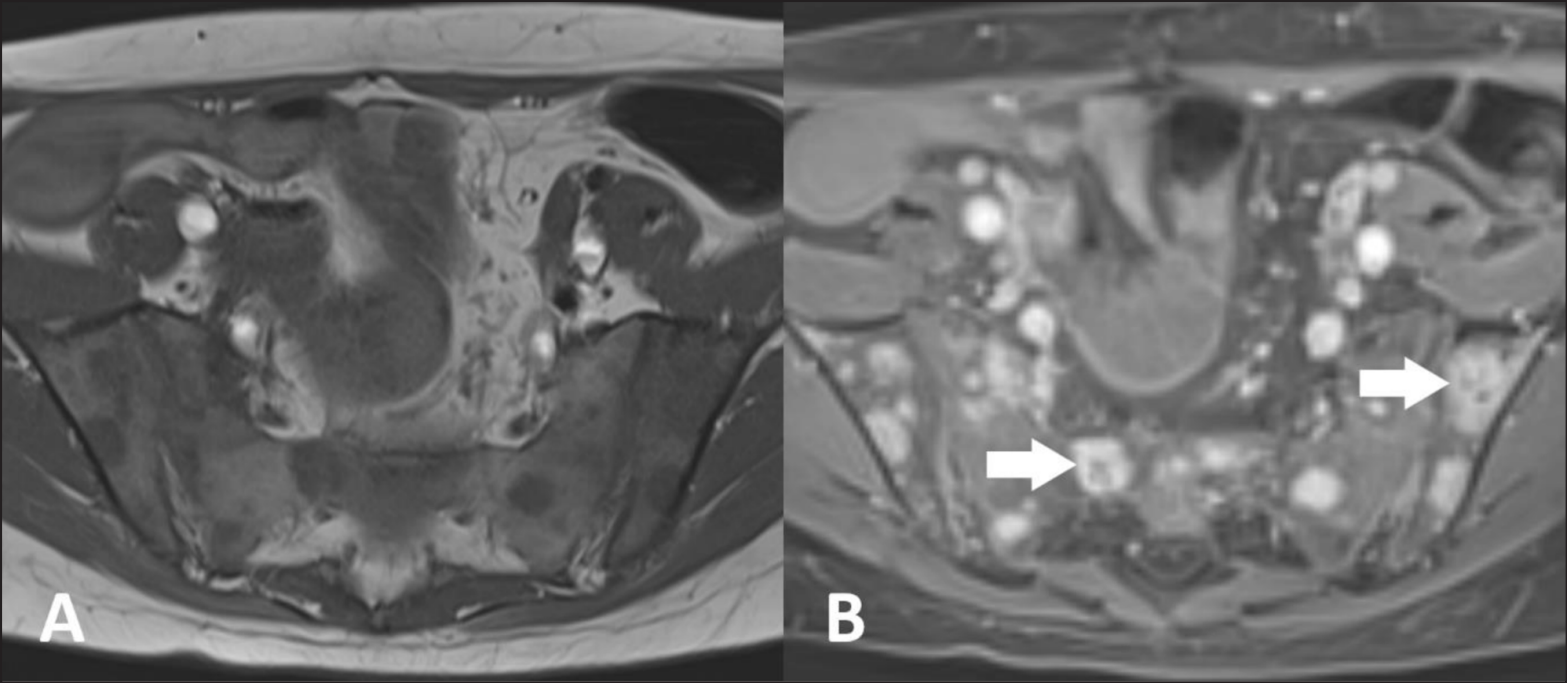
A 13-year-old female with hemangiosarcoma and sacral metastasis. (a) Axial T1-weighted MR-image shows multiple hypointense lesions in sacral and iliac bone. (b) Axial fat-saturated post-contrast T1-weighted MR image shows strong enhancement of the lesions with central necrosis in the largest lesions (arrow).
